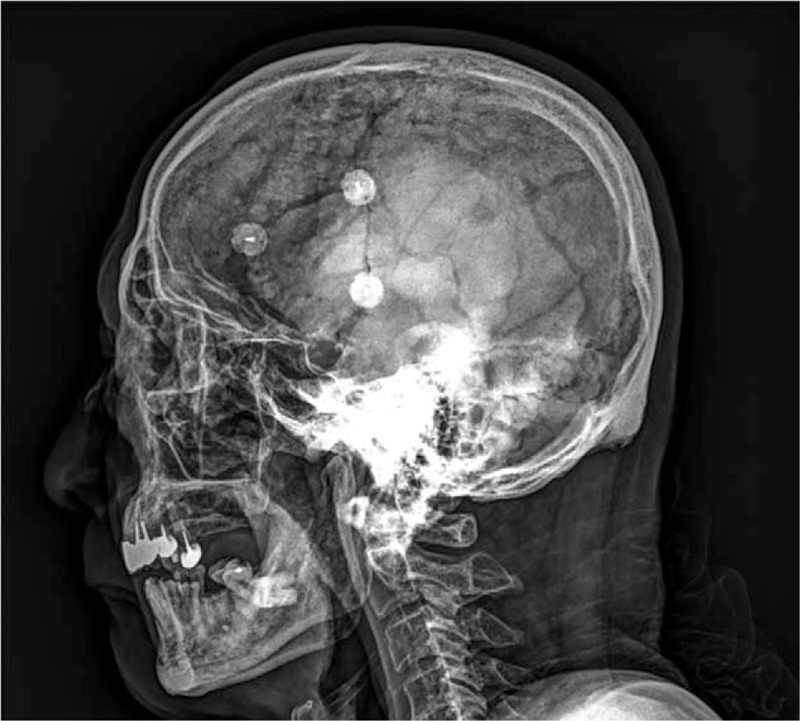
The skull X-ray plain film demonstrated no osteolytic lesions.
